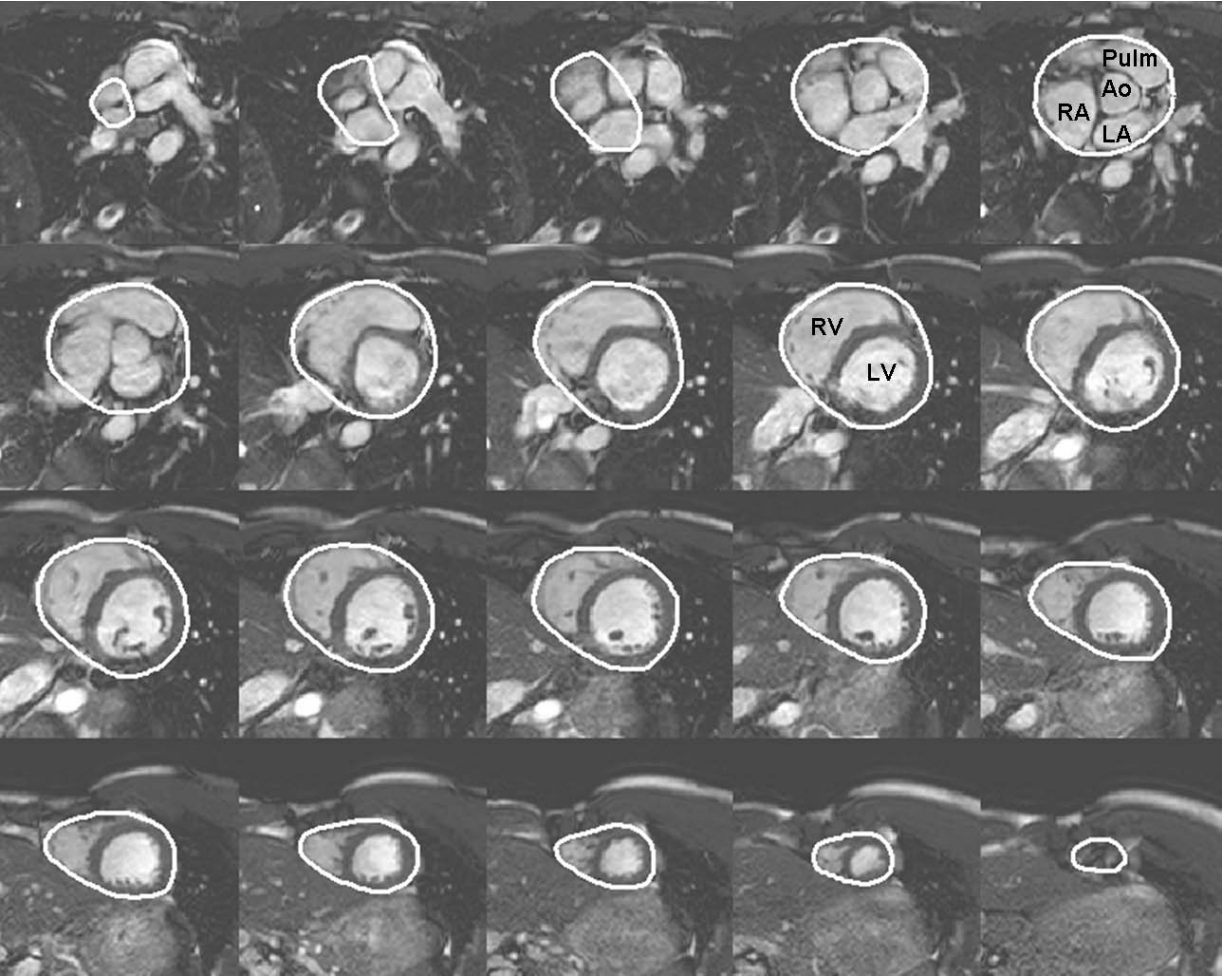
Delineation of total heart volume. Example of delineation of the pericardium from short-axis cardiac magnetic resonance images enabling determination of the total heart volume. The base is seen in the upper left corner and apex in the lower right corner. Ao = ascending aorta, LA = left atrium, LV = left ventricle, Pulm = pulmonary artery, RA = right atrium, RV = right ventricle.
